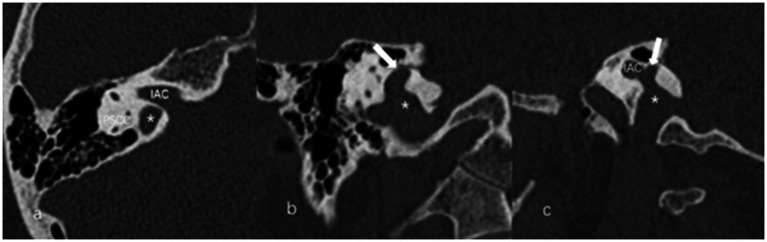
The axial (a), coronal (b), and sagittal (c) images of jugular bulb diverticulum (JBD). (a) High jugular bulb (asterisk) lies in the triangular area between the internal auditory canal (IAC), posterior semicircular canal (PSCC) and posterior surface of the petrous bone. (b,c) Jugular bulb diverticulum is displayed as a vertical outpouching (arrow) from the jugular bulb (asterisk) on the coronal and sagittal image of temporal bone high-resolution computed tomography.
